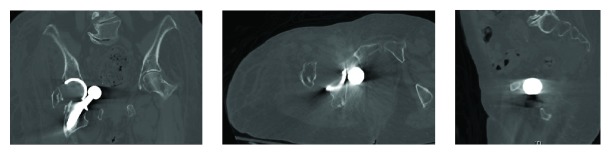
Coronal, axial, and sagittal computed tomography (CT) images showing femoral component dislocation through the right obturator canal and abutting the urinary bladder.
